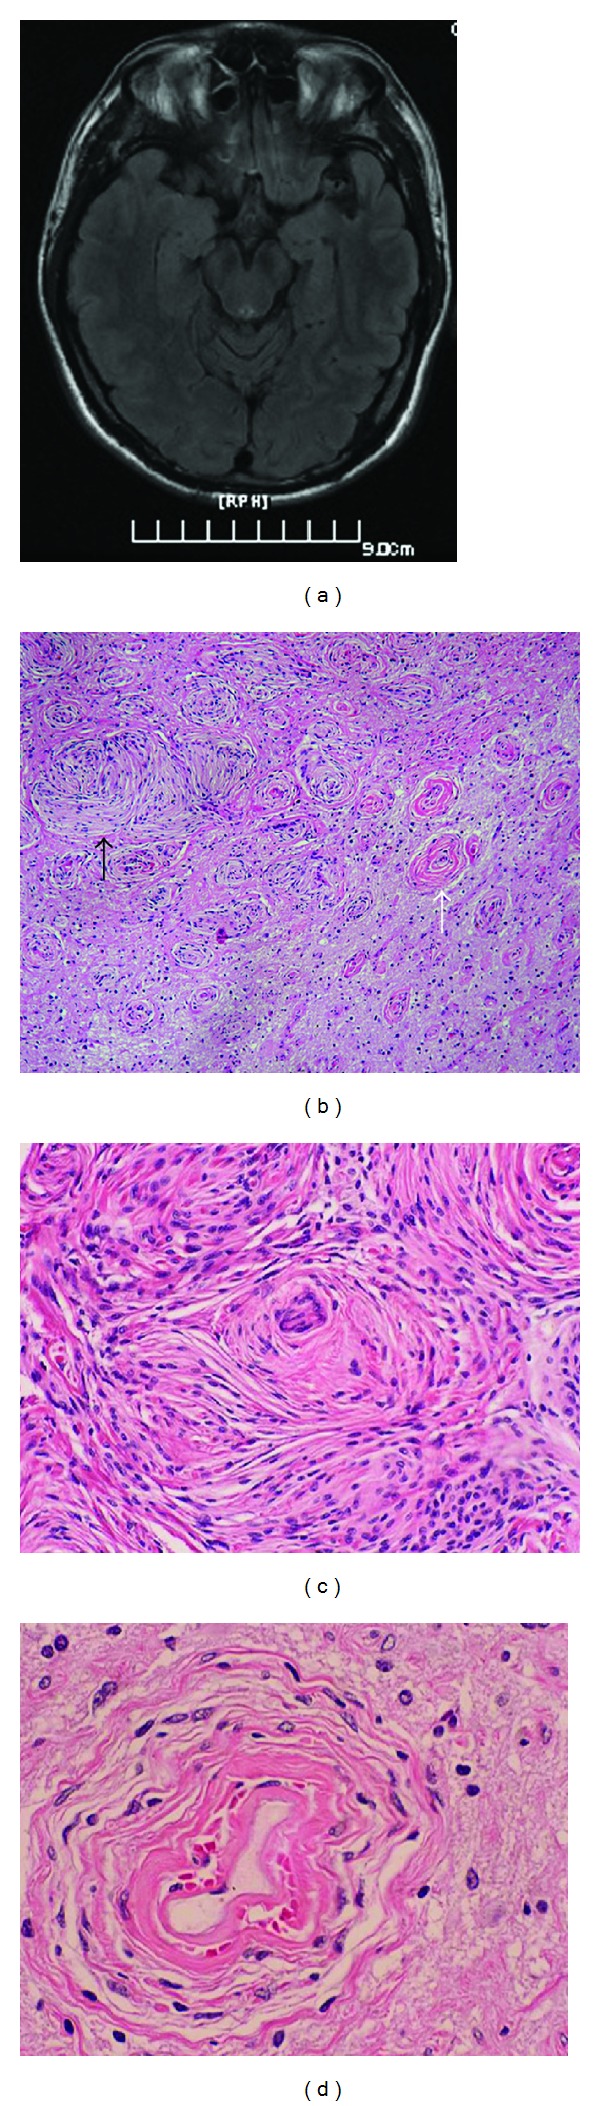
(a) MRI shows a low-signal intensity in the left temporal lobe on T1-weighted image; (b) transition area between MA (white arrow) and meningioma (black arrow), HE ×40; (c) transitional meningioma, HE ×200; (d) MA area is typical of collagenized vessels cuffed by meningothelial cells or fibroblasts under high power (HE ×400).
